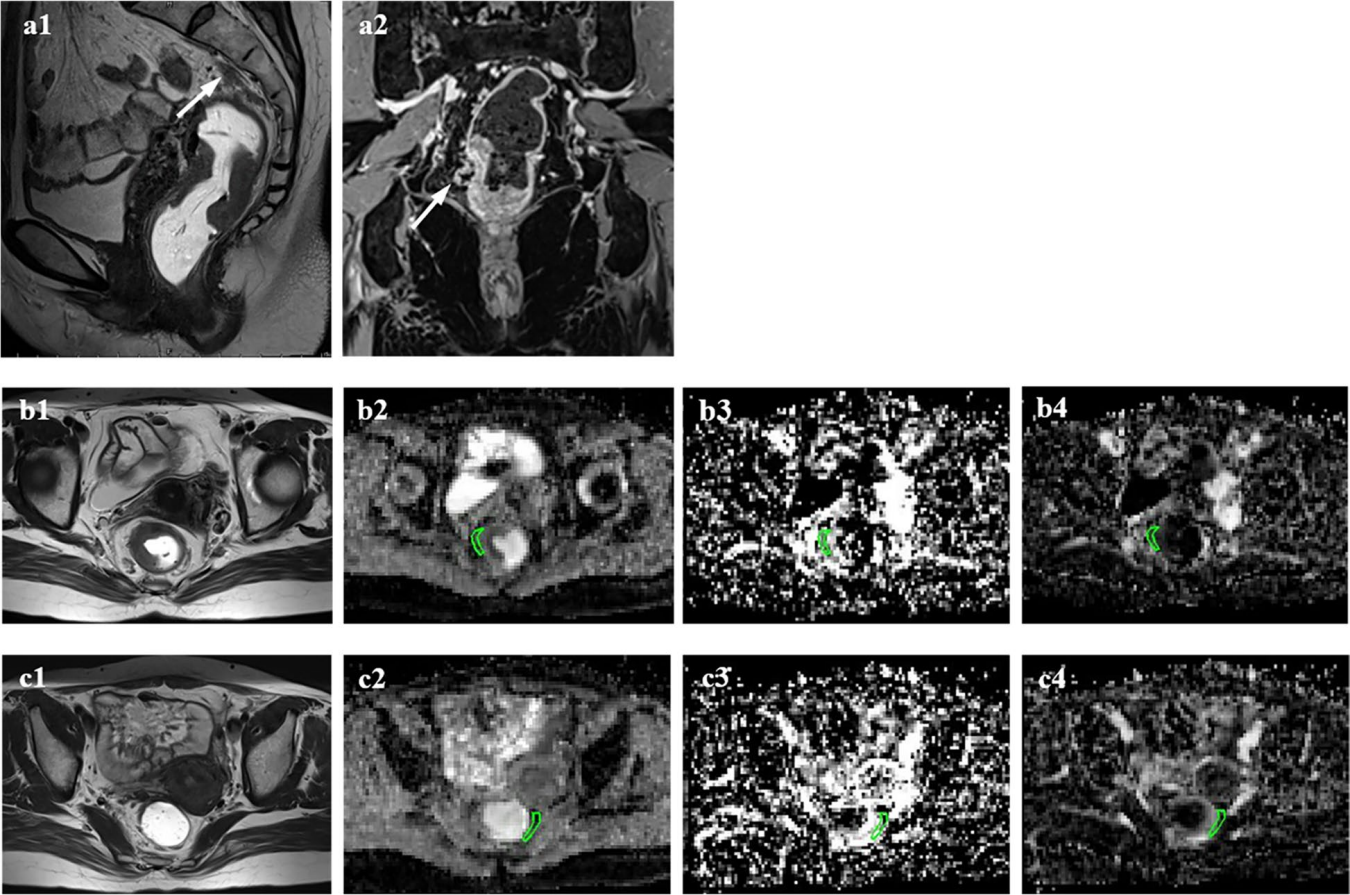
Measurement of IVIM parameters in the mesorectum in patients with positive mrEMVI. Sagittal T2-weighted imaging (a1) shows a tubular structure that contains a signal void (arrow). On coronal contrast-enhanced T1-weigheted imaging (a2), a small serpiginous vessel outside the tumour demonstrates an irregularly expanded contour (arrow). The ROIs in the MAT (b2 – b4) are located within 5 mm of the outline of the largest tumour segment, and the calculated IVIM parameters included D (1.45 × 10^–3^ mm^2^/s), D* (10.79 × 10^–3^ mm^2^/s), and *f* (0.15). The ROIs in the MDT (c2 – c4) are located contralateral to the tumour parenchyma as far as possible; D = 0.67 × 10^–3^ mm^2^/s, D* = 12.25 × 10^–3^ mm.^2^/s, and *f* = 0.34
